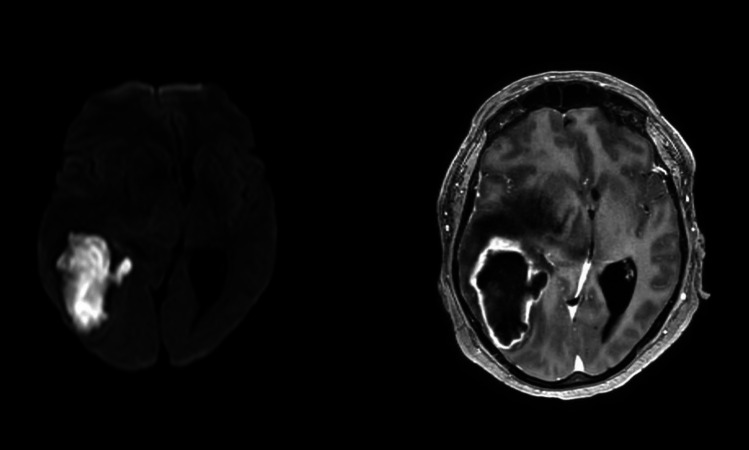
Visualization of an brain abscess by MRI. Diffusion weighted Imaging (left) demonstrating restriction of diffusion, T1 sequence with Gadolinium (right) demonstrating a large abscess with enhancement of the abscess capsule and perilesional edema leading to midline shift
